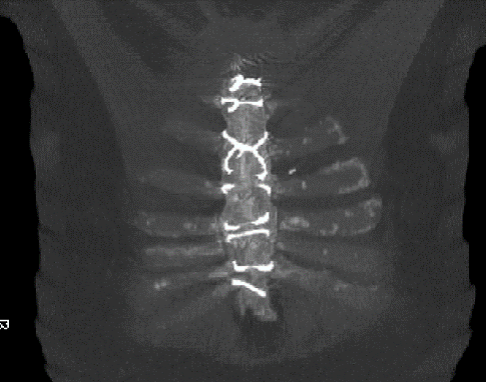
Coronal computed tomography (CT) scan demonstrating the complete osseous wound healing following three months of wearing a compression garment vest (7 months after operative refixation).
